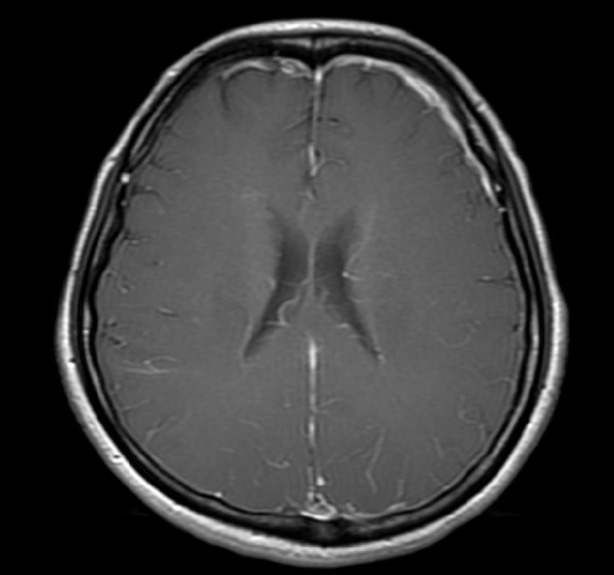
Axial T1-weighted view of cranial MRI after intravenous administration of gadolinium Pachymeningeal enhancement at the bilateral cerebral convexity with more irregular dural thickening at the left frontal convexity is demonstrated. This enhancement is due to small, thin-walled dilated blood vessels in the subdural zone
